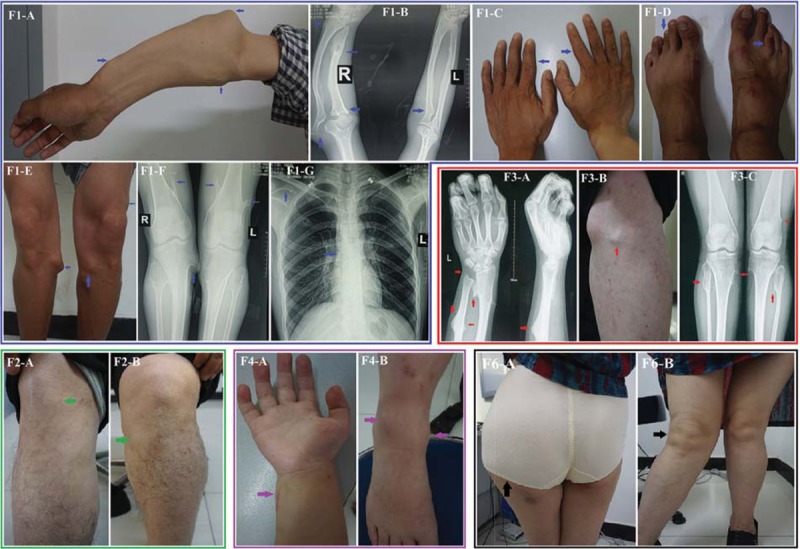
Appearance and radiology results of the probands. F1: (A–G) Obvious right forearm deformities (protruding from the upper lateral radius, forearms bend inward), left ulnar tuberosity bulges (F1-A), a relatively truncated and curved ulna and radius (F1-B), short metacarpals (F1-C), third brachymetatarsia (F1-D), multiple exostoses around the knees (F1-E), localized bony protrusions on the distal femur, proximal tibia and fibula (F1-F), mild symptoms of costal and scapular exostoses and scoliosis (F1-G) were observed in the proband (V: 2) and are indicated with blue arrows. F2: (A, B) Exostoses that occurred around both knees (F2-A, F2-B) were found in the proband (III:2) and are indicated with green arrows. These exostoses had been partly surgically removed. F3: (A–C) X-rays showed multiple bony protrusions in the left distal radius and ulna (F3-A), as well as exostoses around both knees (F3-B). X-rays showed localized bony protrusions around both knee joints and slightly narrowed joint spaces (F3-C) (proband: III:1, indicated with red arrows). F4: (A, B) Exostoses at multiple sites, including the right bottom of the radius and wrist (F4-A) and the right side of the lower tibia (F4-B) (proband: III:1, indicated with purple arrows). F6: (A, B) Exostoses that were located on the upper side of the left femur (F6-A), the coxa valga and mild scoliosis were observed in the proband (II: 4, F6) (F6-B) (proband: II: 4, indicated with black arrows).
